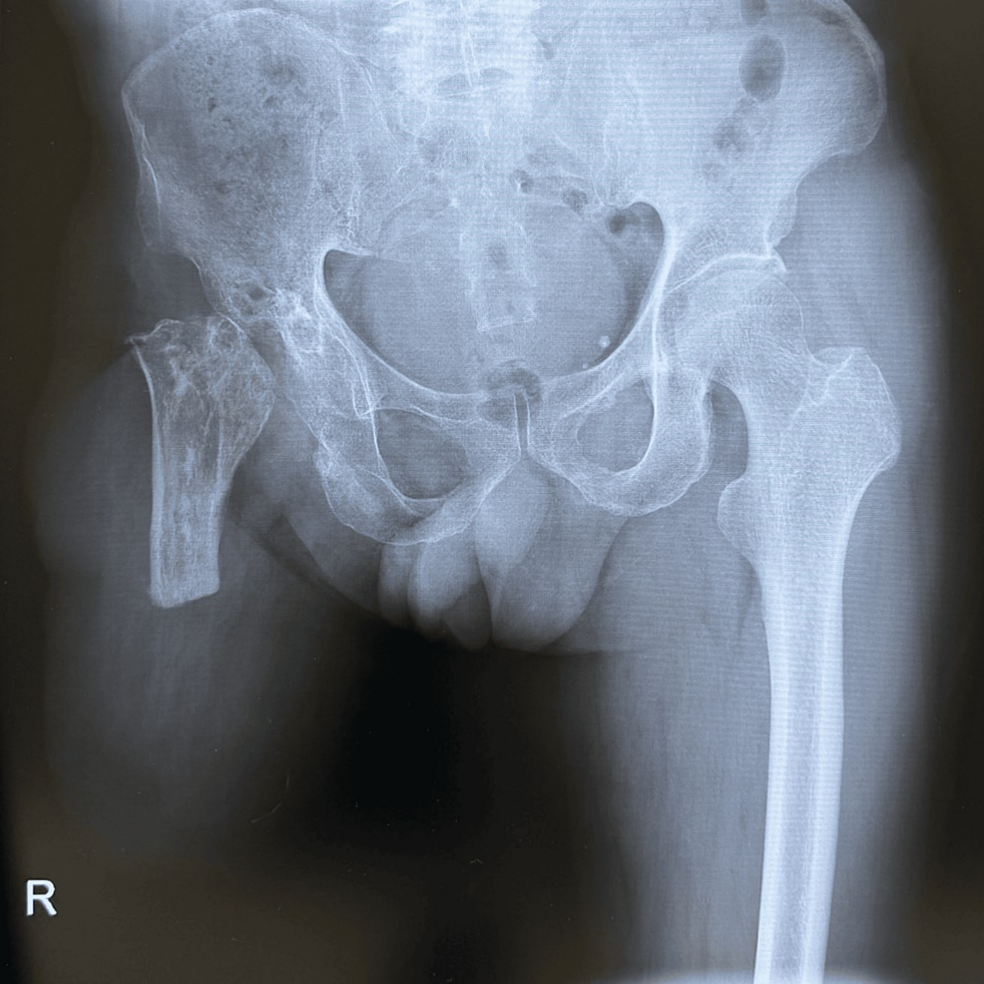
Anteroposterior radiograph of the right Girdlestone hip and transfemoral amputation
